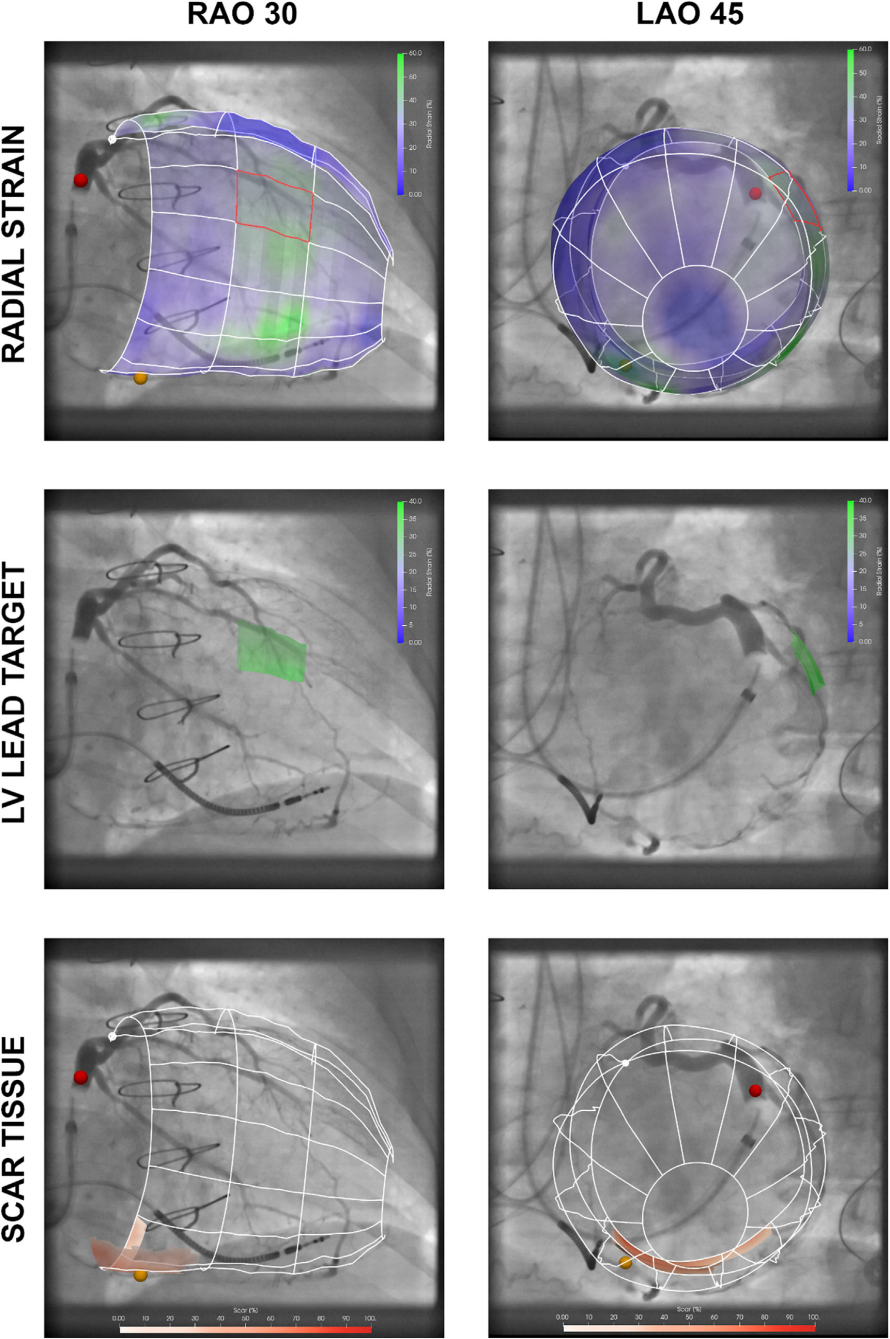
Model-to-image registration to guide left ventricular (LV) lead implantation in real time. The 36-segment mesh is derived from cardiac magnetic resonance imaging and is superimposed on dual-view fluoroscopic venograms, using the right anterior oblique (RAO) (left column) and left anterior oblique (LAO) (right column) acquisitions. Sites of latest mechanical activation (green, upper panels) are targeted and scar tissue (red, lower panels) is avoided. In this case, the most suitable target for lead implantation is determined as mid-lateral 1 and displayed to the implanting physician (middle panel). The red dot indicates the coronary sinus and the orange dot the middle cardiac vein.
